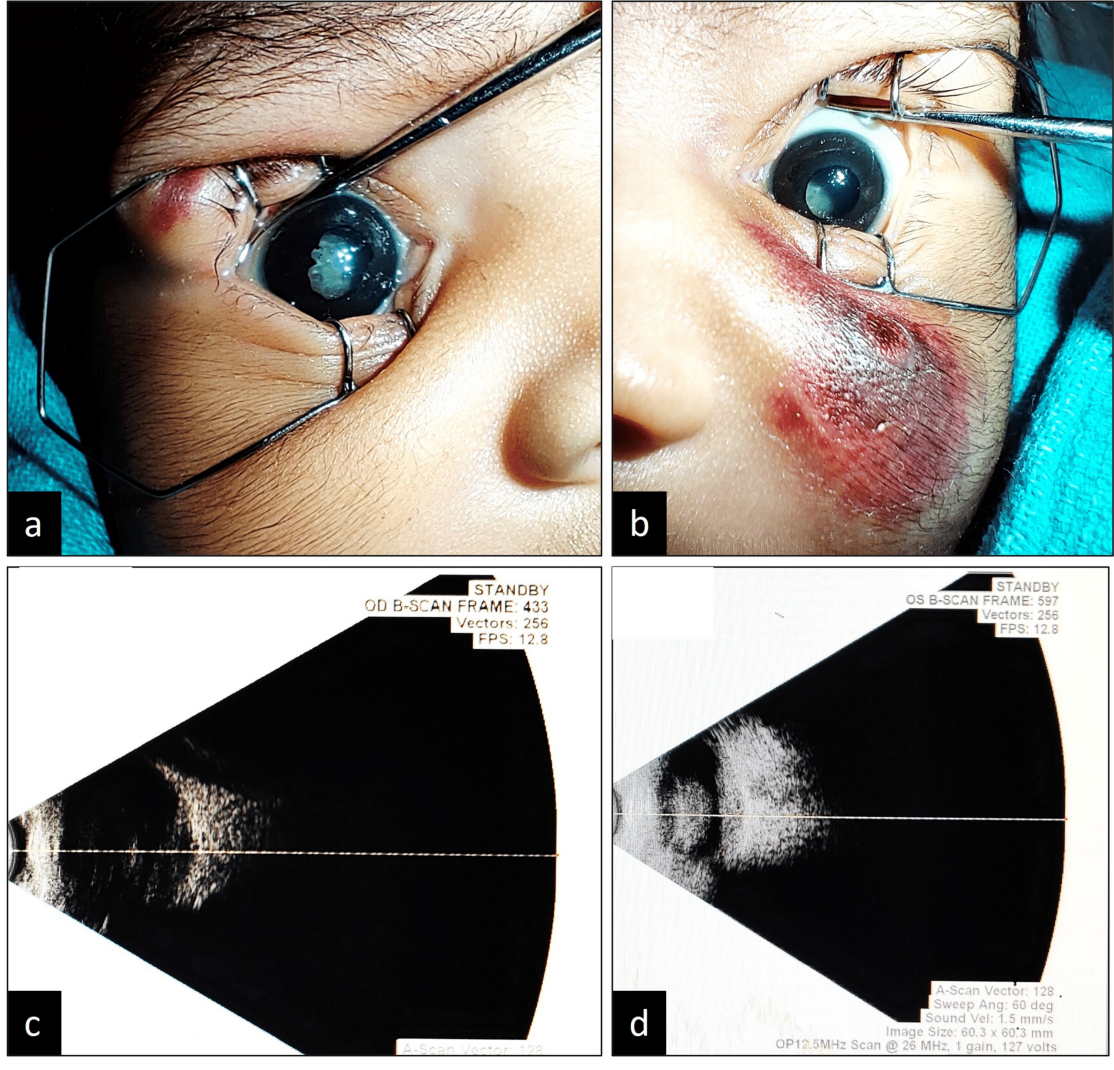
Bilateral white pupillary reflexes and pupillary synechiae (a, b). Ultrasound B-scan demonstrates moderate-to-high-intensity echoes suggestive of vitreous hemorrhage and retinal detachment (c, d).
